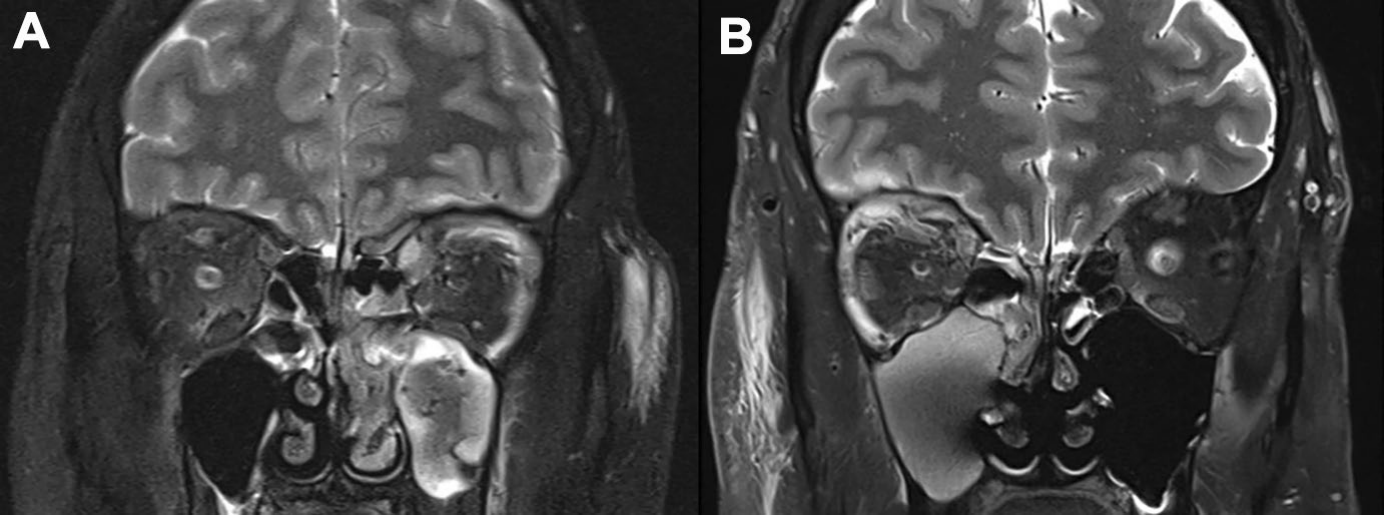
T2-weighted fat-suppressed MRI demonstrating heterogeneous hyperintensity in the region of concern. A and B demonstrate superolateral extraconal inflammatory changes in the left (Patient 1) and right orbits (Patient 4), respectively
